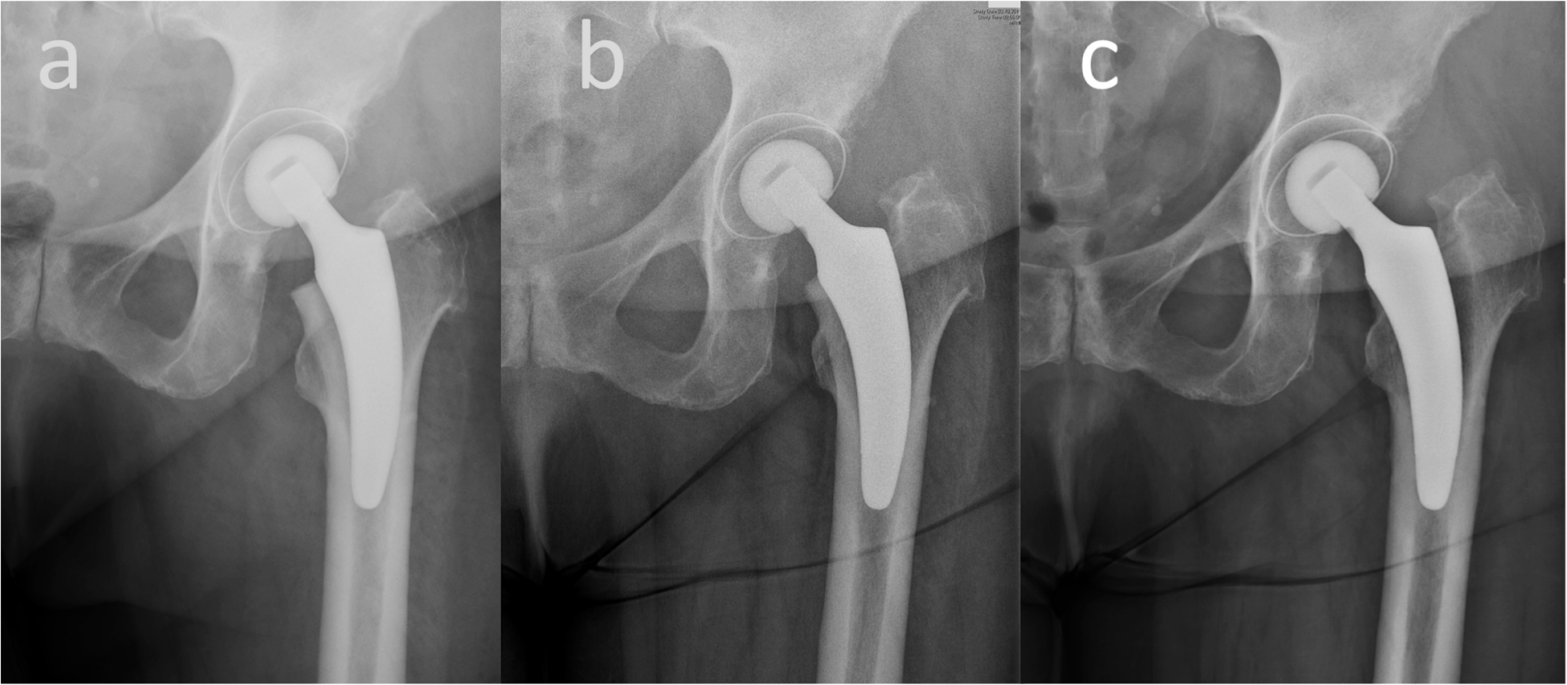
Aseptic loosening of the stem in one patient. The x-ray images of the case, in which an aseptic loosening was diagnosed is depicted. a Postoperative, b 3 months and c 6 months postoperative images are presented
